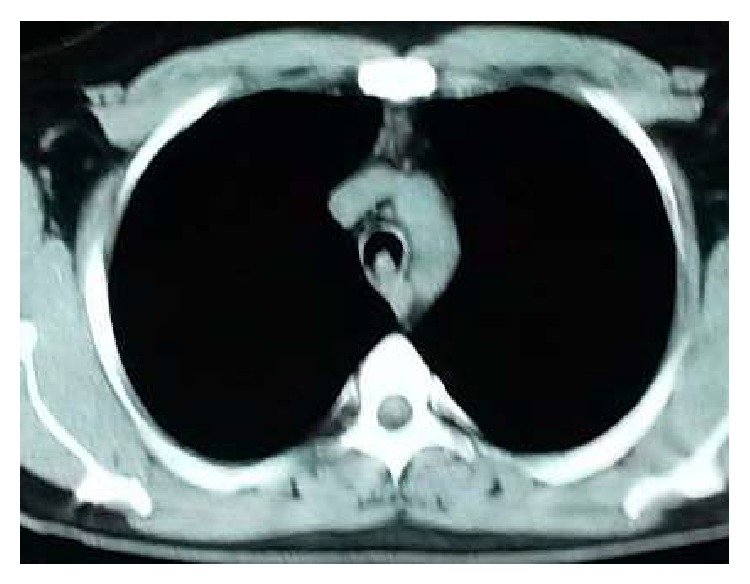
Chest CT showed a lesion protruding to the lumen in distal trachea at the posterior wall.
